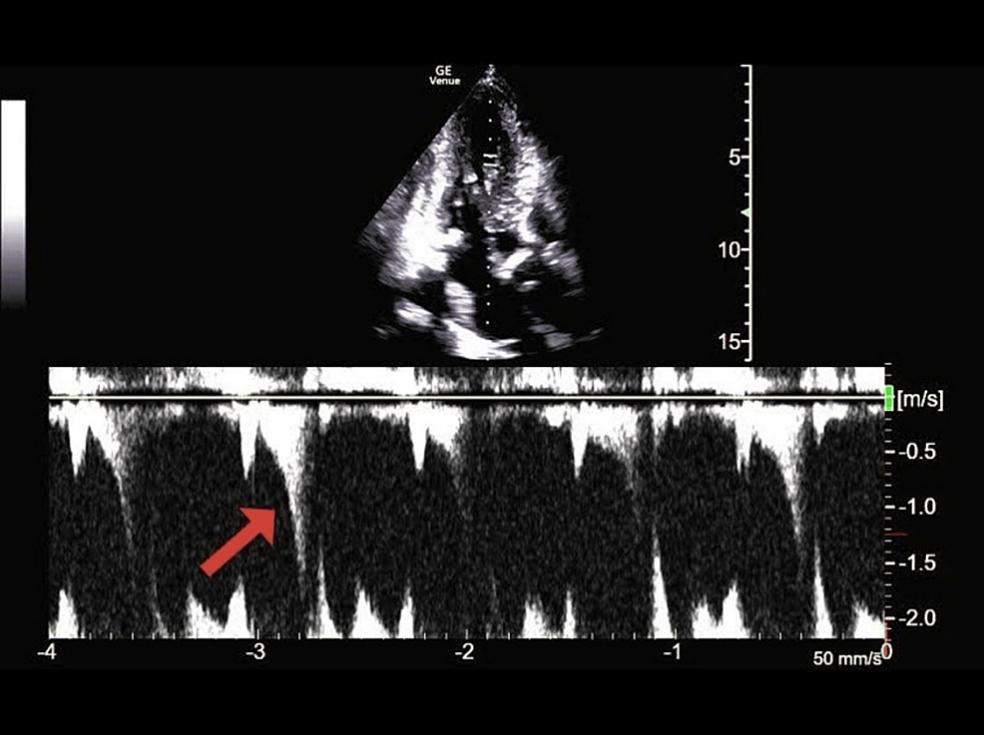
Pulsed-wave Doppler of the mid-LV cavity revealing a late-peaking, scythe-shaped velocity envelope LV: Left ventricle.
